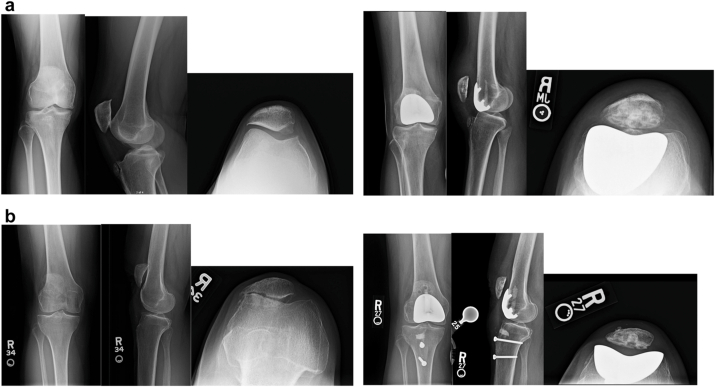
Representative preoperative and postoperative radiographs: (a) patellofemoral arthroplasty (PFA) with medial patellofemoral ligament (MPFL) reconstruction, (b) patellofemoral arthroplasty (PFA) with tibial tubercle osteotomy (TTO).
